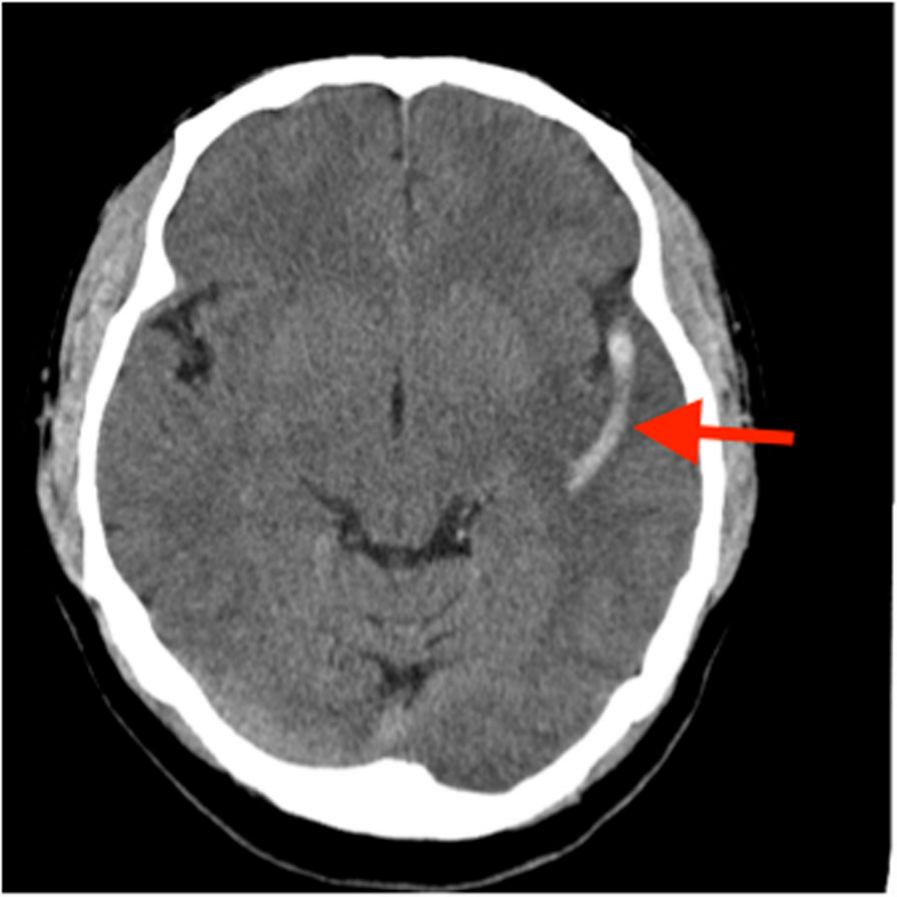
A brain computed tomography image showing the thrombus in the left middle cerebral artery (arrow)
